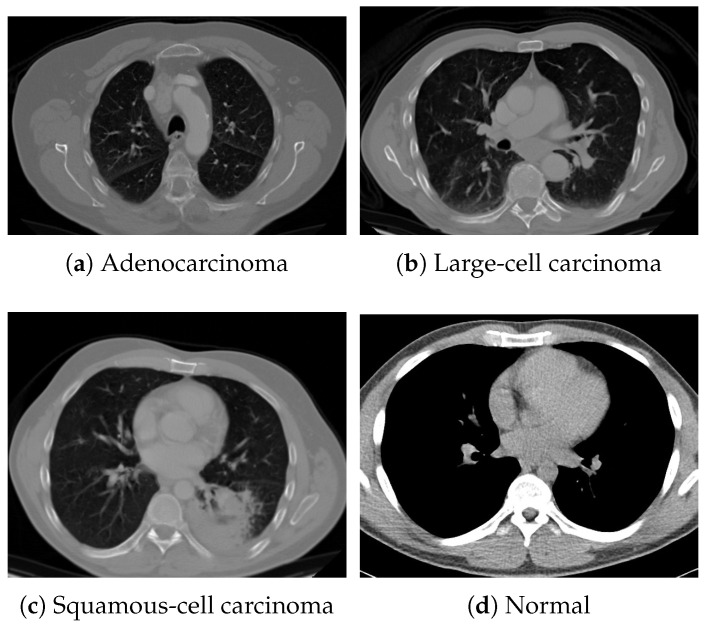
Examples of chest CT images from the Chest CT-Scan Images dataset: (a) adenocarcinoma, (b) large-cell carcinoma, (c) squamous-cell carcinoma, and (d) normal.
